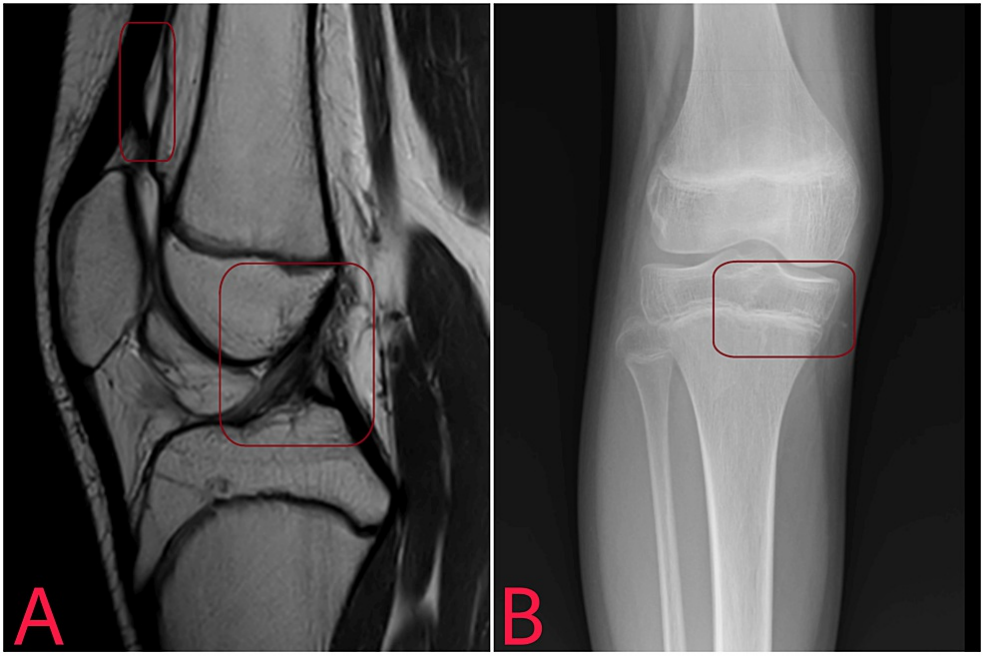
An MRI (A) showing a healed suprapatellar lipohemarthrosis and anterior cruciate ligament. An X-ray (B) showing a reunited epiphyseal plate of the proximal tibia.
